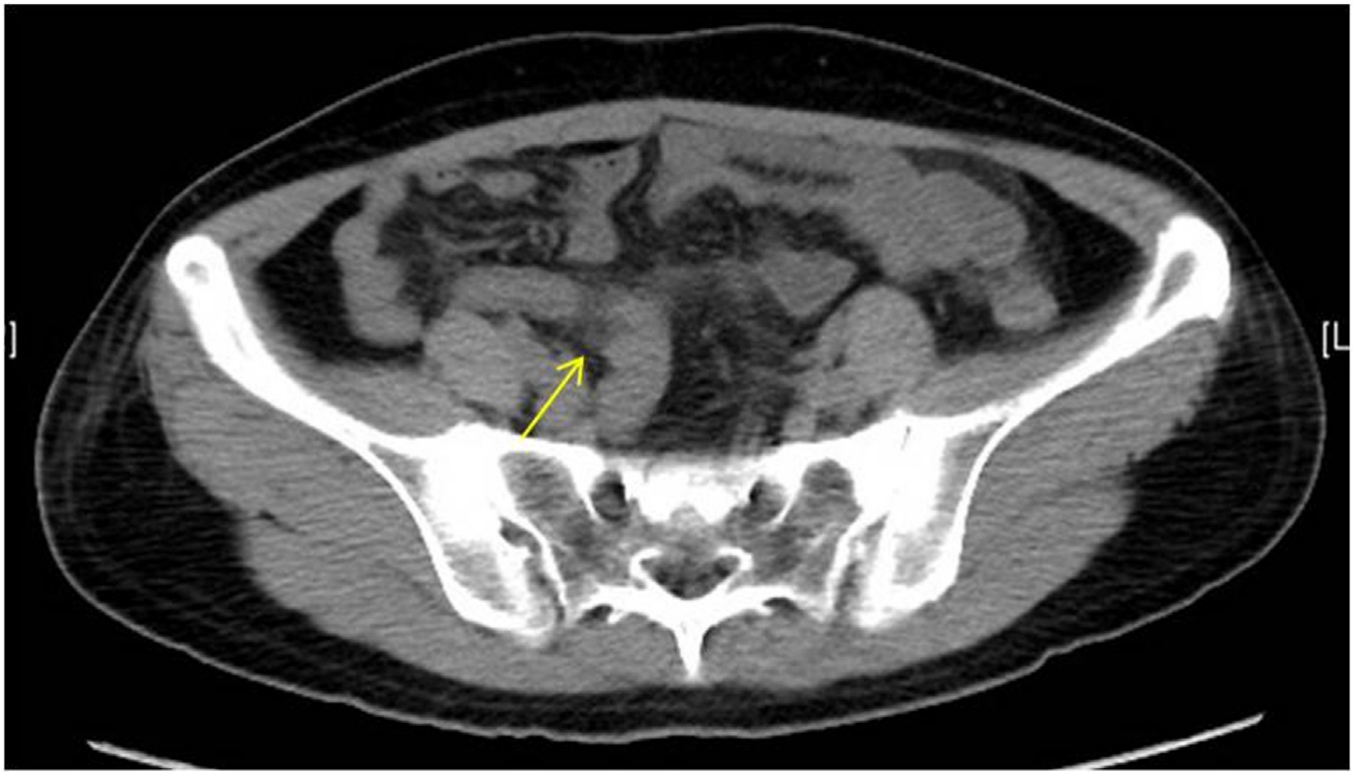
Noncontrast axial computed tomography of the abdomen showing increased appendiceal outer-to-outer diameter of 8.7 mm, wall thickening, and periappendiceal inflammation (*yellow arrow*). No evidence of free air or fluid to suggest perforation is observed.
